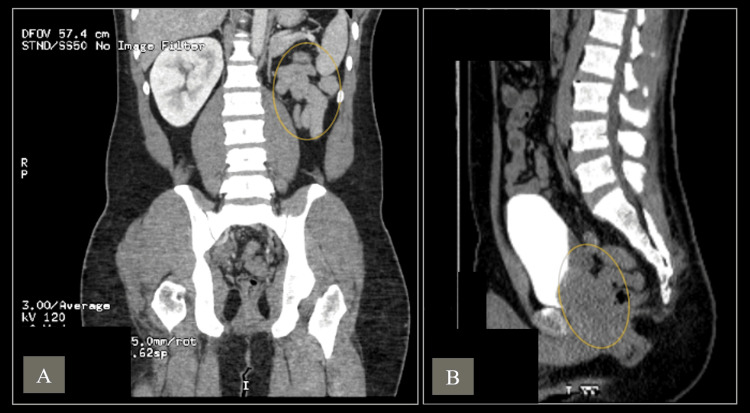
Contrast-enhanced CT of the abdomen and pelvis demonstrating congenital left renal agenesis and a large seminal vesicle cyst measuring 7 × 5 cm. (A) Coronal view. The yellow circle highlights the congenital absence of the left kidney (renal agenesis). (B) Sagittal view. The yellow circle highlights the 7 × 5 cm left seminal vesicle cyst.
